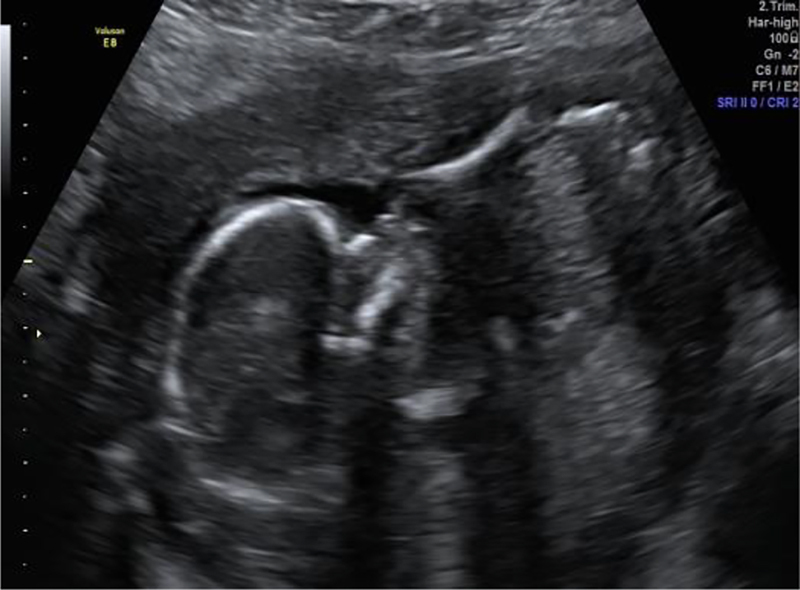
Ultrasound at 18 weeks showing anhydramnios.
